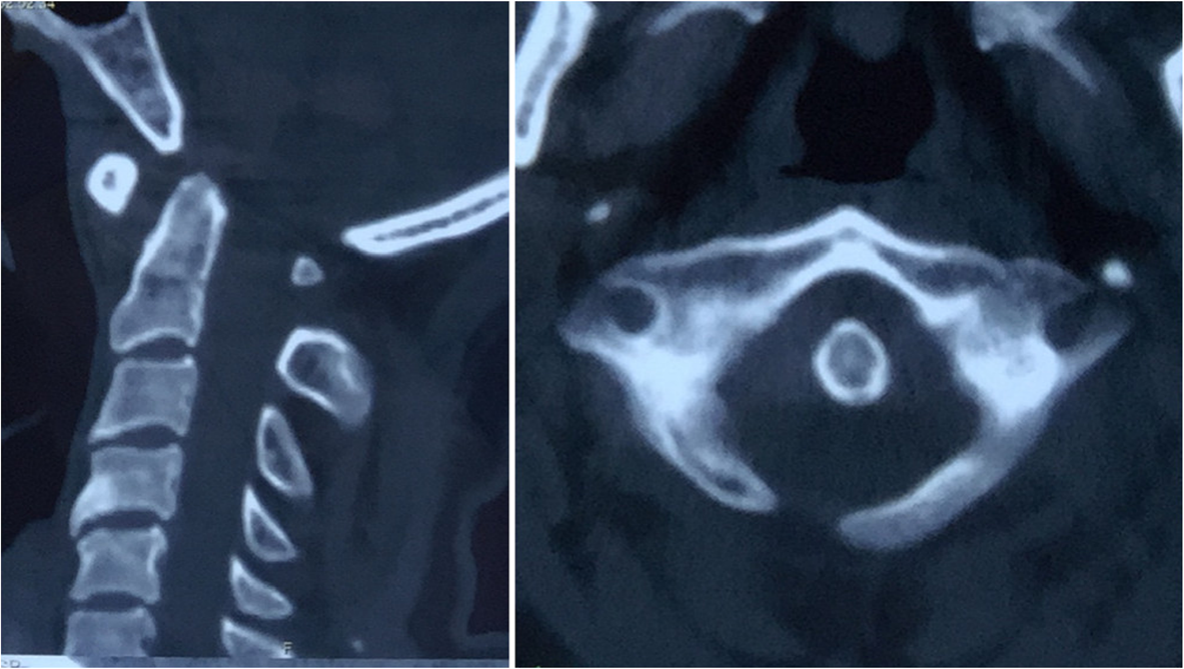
Computed tomography (CT) scan revealed obvious anterior atlantoaxial dislocation
